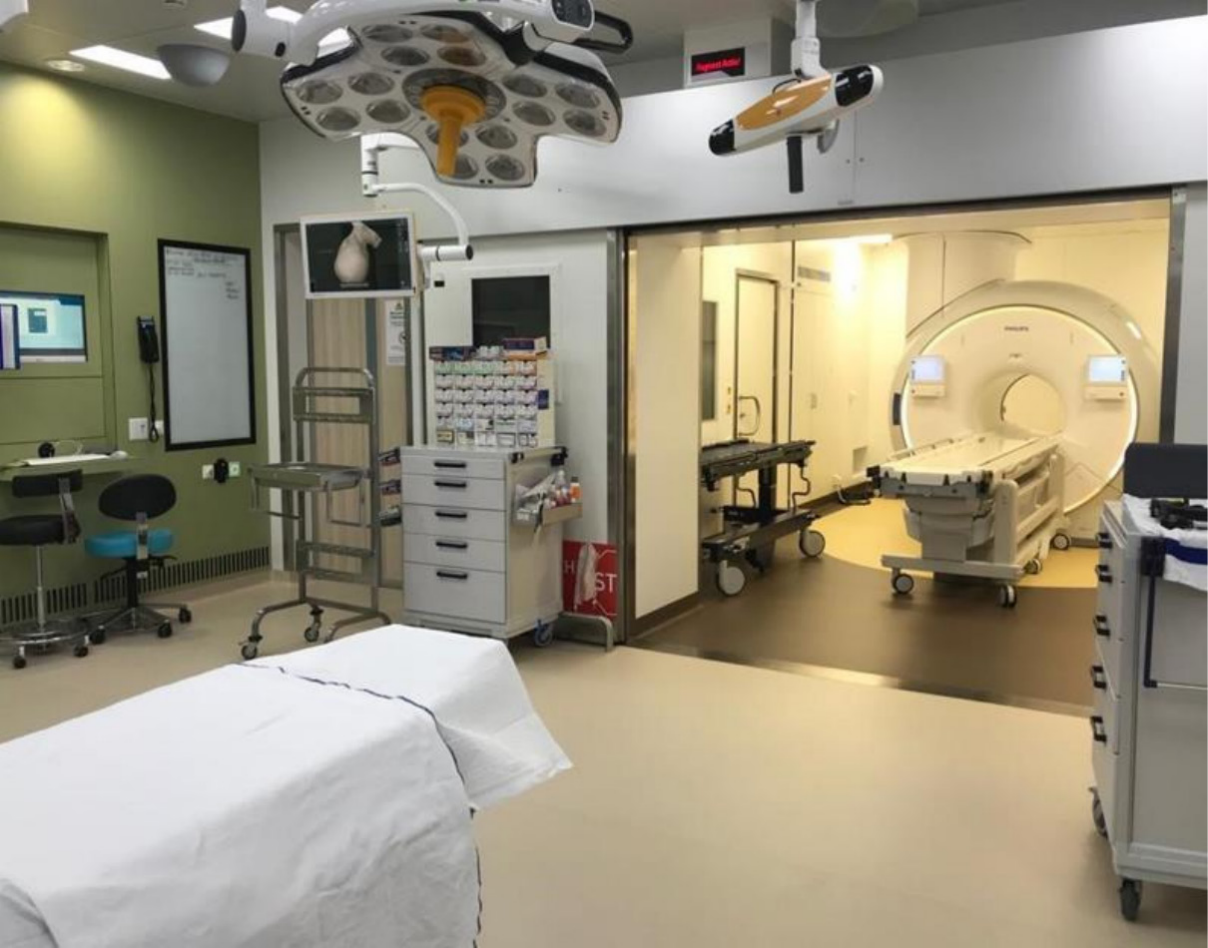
Set up of the intraoperative MRI operating theatre.
